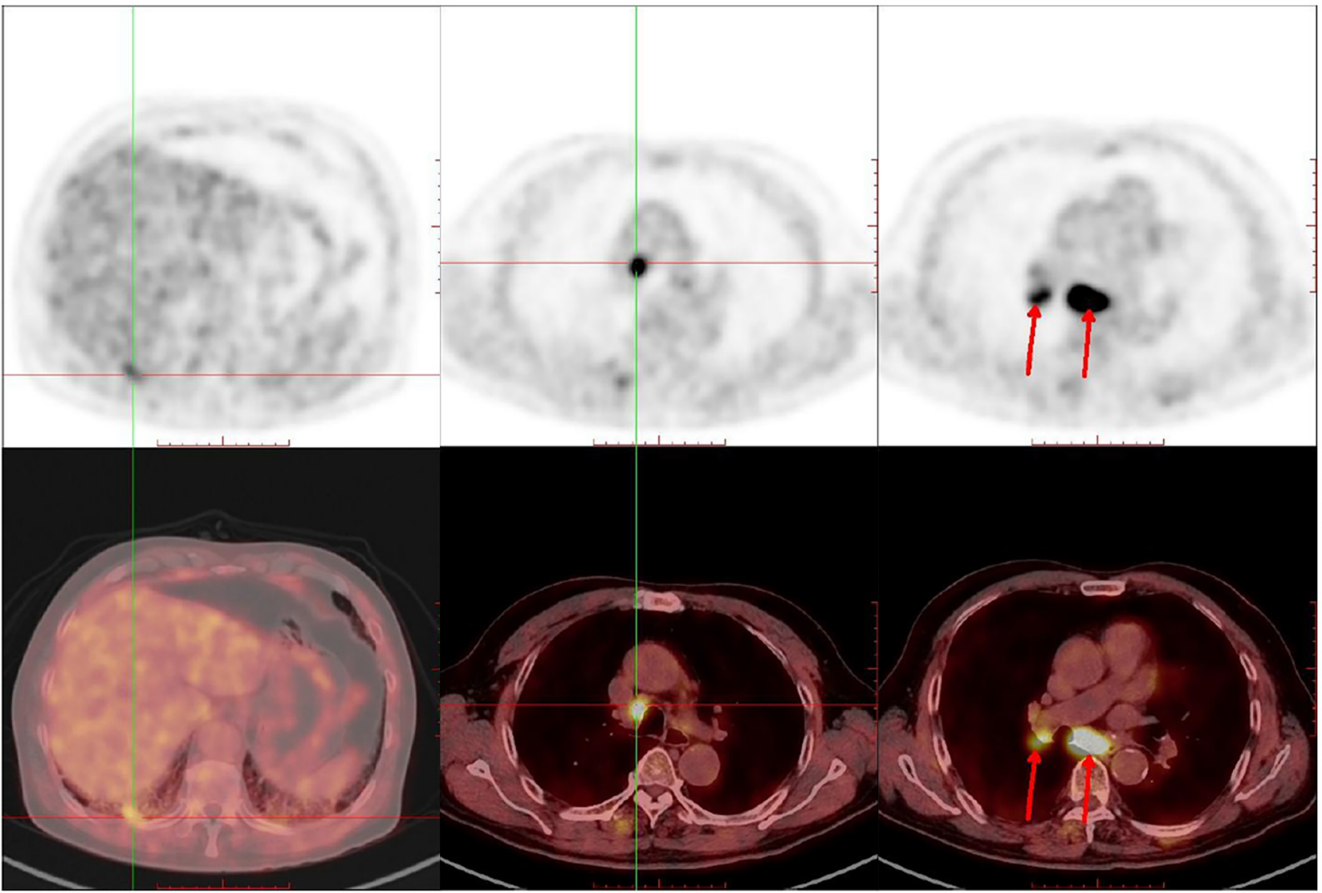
PET/CT revealed a right lower lobe mass and 4R/7 lymphadenopathy before treatment.
